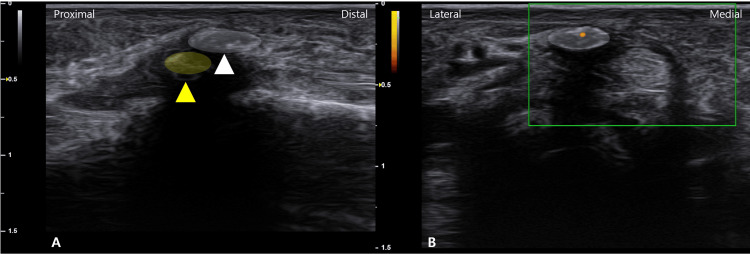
Ultrasound findings of the calcification of the A1 pulley. (A) Calcification (white arrowhead, white shaded area) is observed separately from the sesamoid bone (yellow arrowhead) on a long-axis image. (B) Signal changes are observed on power Doppler.
